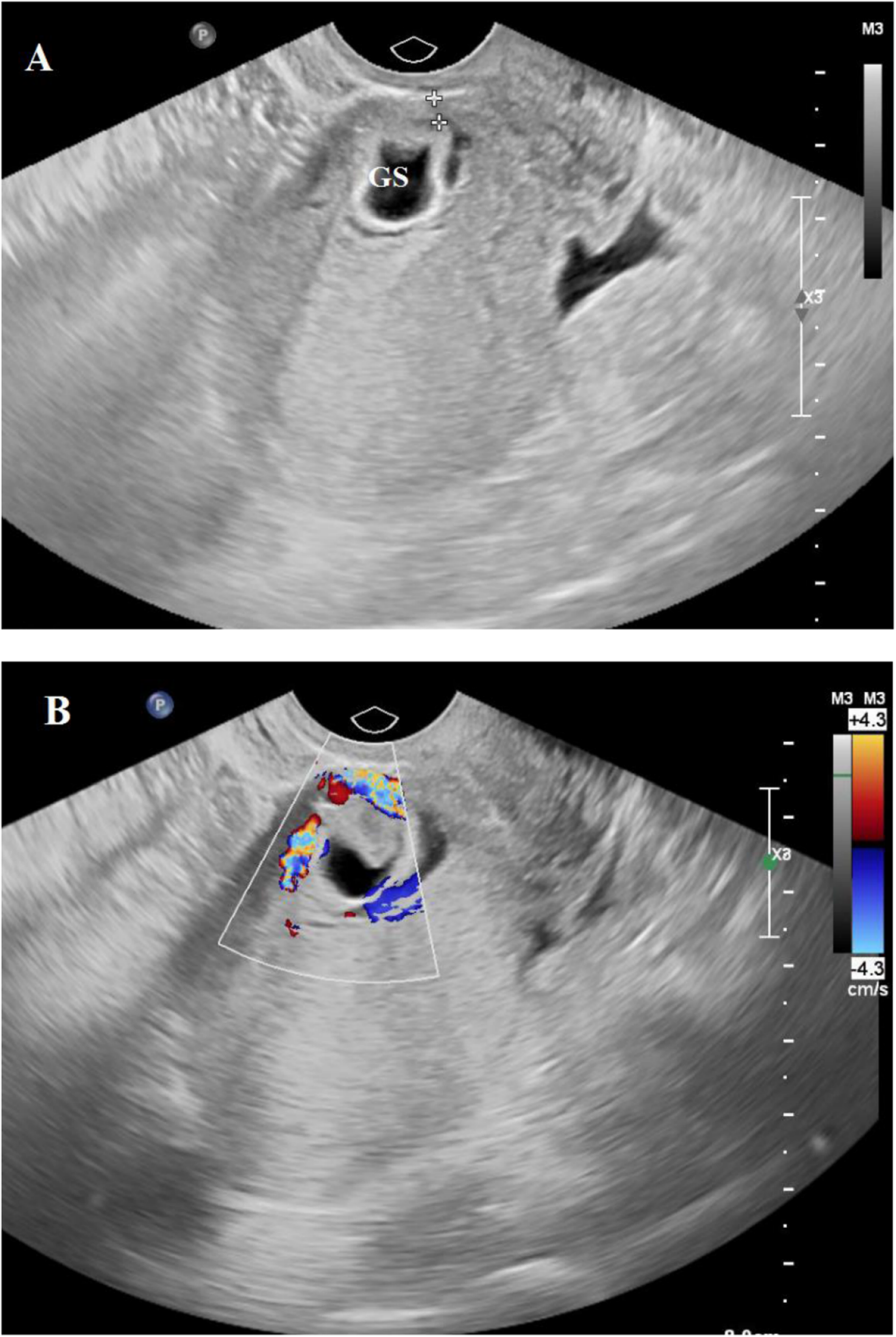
Transabdominal ultrasound (a) of a patient with caesarean scar pregnancy showed an empty uterine cavity and cervical canal, and the gestational sac (GS) implanted at the site of a cesarean scar and surrounded by thin myometrium. The Doppler ultrasound (b) revealed the increased vascular flow surrounding the GS
